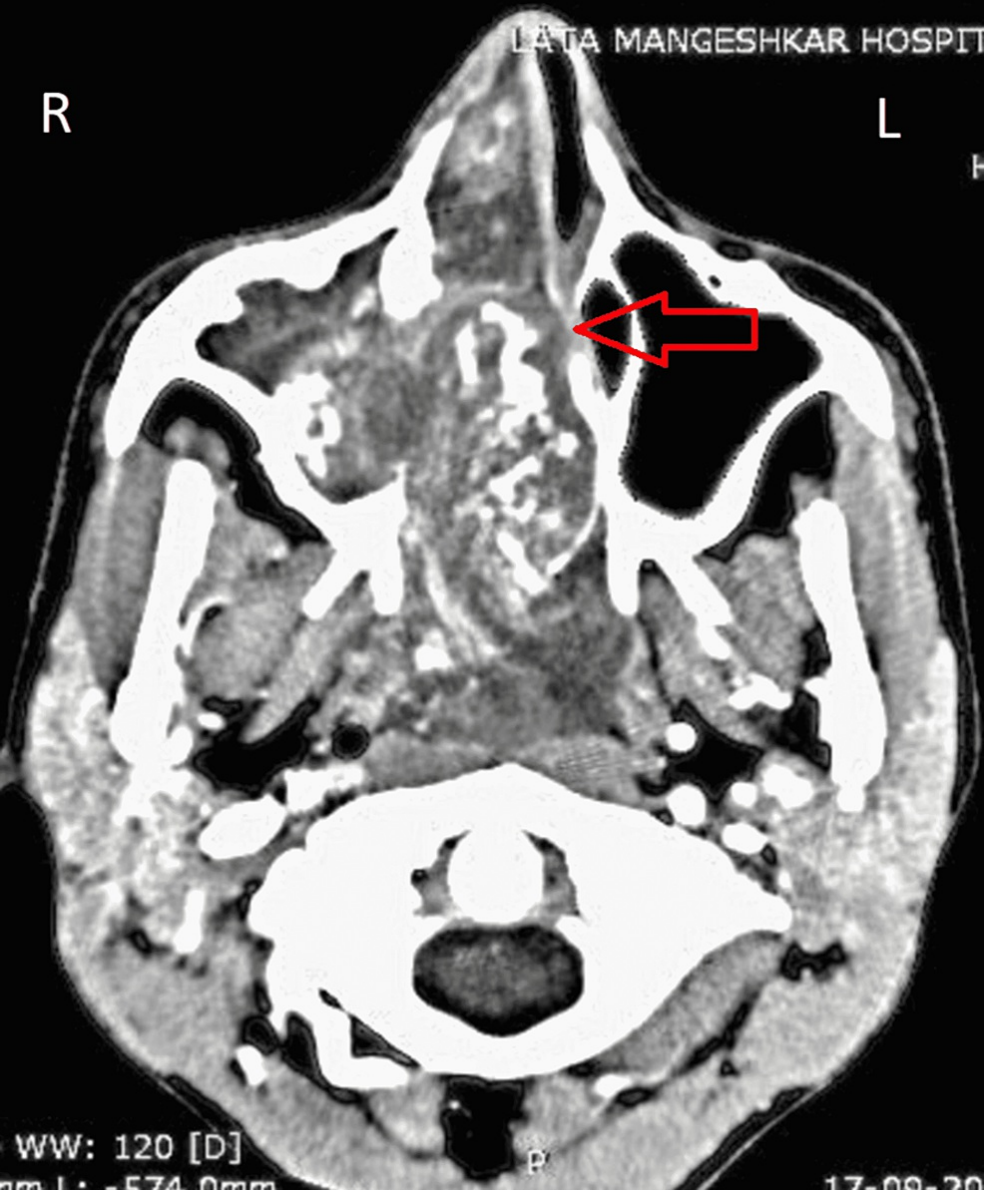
Post-contrast axial plane CT-PNS image showing heterogeneous intense enhancement.
